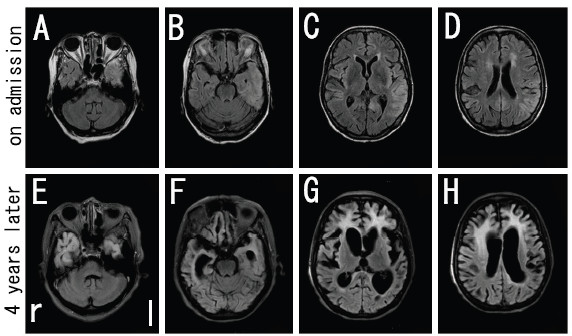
FLAIR images of brain MRI in the present case. Increased signal intensity was observed on admission (A-D). Note that the high-signal region spread to the occipital cerebral cortex and the frontal white matter at four years after the onset with a marked ventricular dilatation (E-H).
